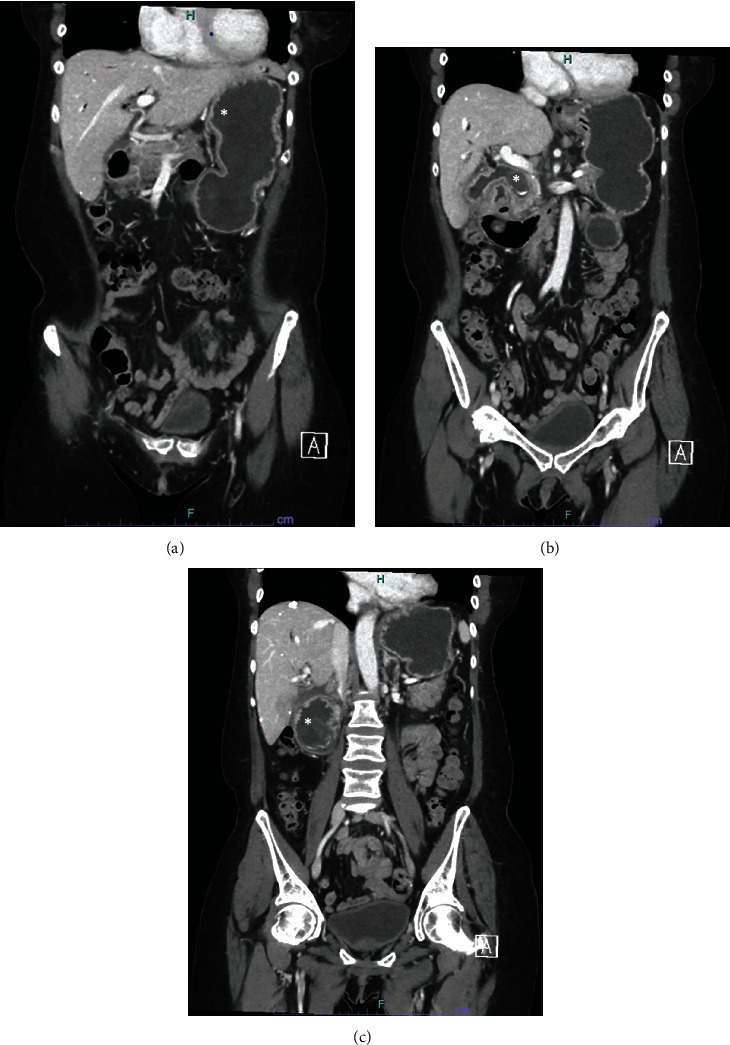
(a) CT A/P from OSH. The asterisk marks the dilated stomach from gastric outlet obstruction. (b) CT A/P from OSH. The asterisk marks the location of a 2.5 cm gallstone in the CBD. (c) CT A/P from OSH. The asterisk marks the location of a 3.5 cm gallstone in the 2^nd^ portion of duodenum.
